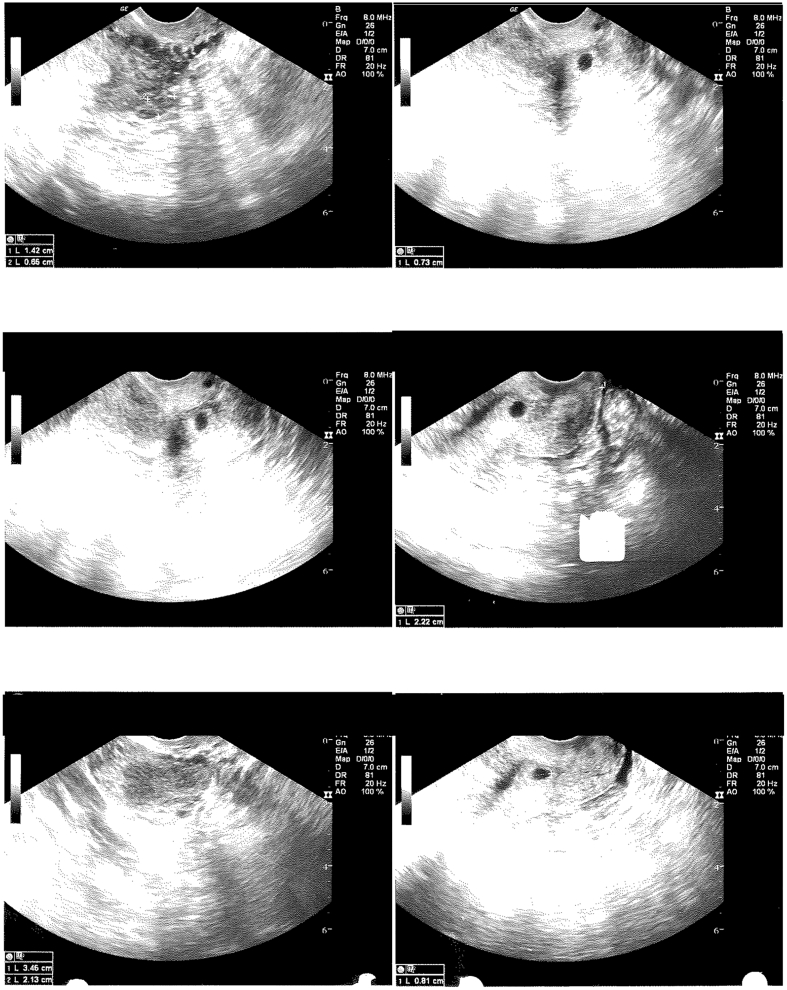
Transvaginal ultrasound at 5 weeks and 1 day of gestation. Gestational sac measuring 8.1 mm in the lower uterine segment versus the upper cervix. Gestational sac measuring 2.2 cm from the distal end of the cervix. No yolk sac or fetal pole visualized. Beta-hCG 3217 mIU/mL.
